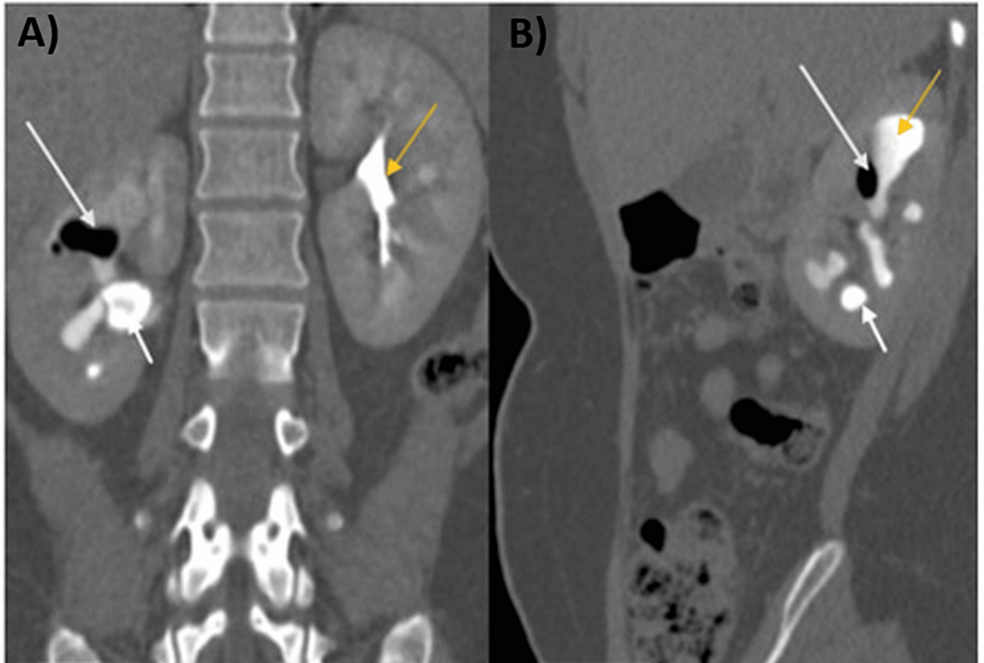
Post-contrast CT images Coronal (A) and sagittal (B) post-contrast CT images show contrast in the pelvicalyceal system (A and B, yellow arrows), multiple air foci in the upper and interpolar calyces (A and B, long arrows), and calculus in the same region (A and B, short arrows).
